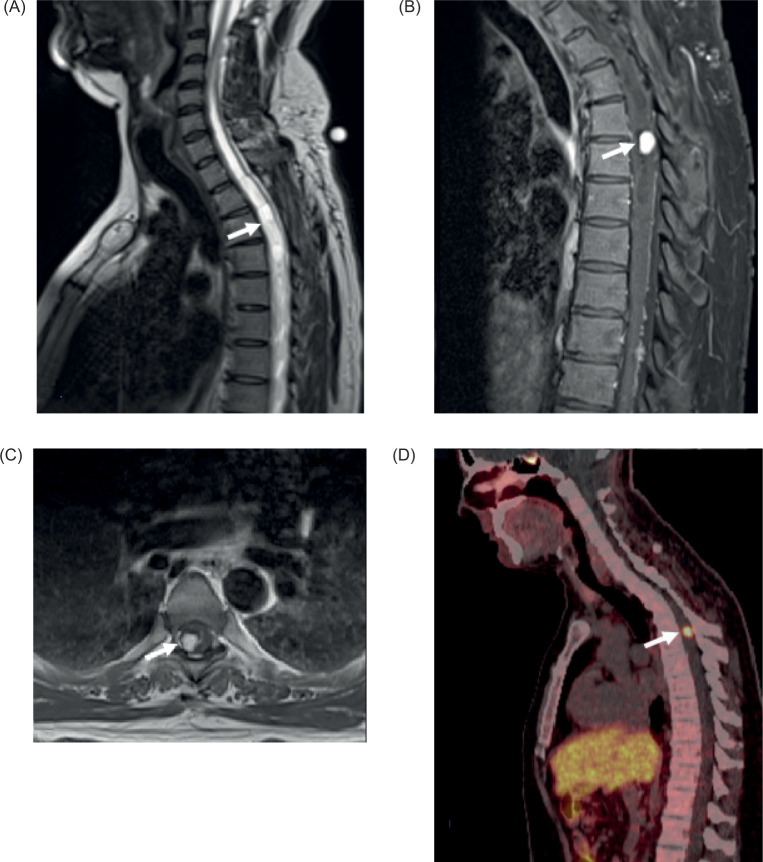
(A) Sagittal T2 image of the thoracic spine shows a long syringohydromyeliain of the cervicothoracic spine (arrow); (B) Sagittal T1 fat sat post contrast and (C) axial T1 post contrast demonstrates an avidly enhancing nodule within the thoracic spine, located within the syringohydromyelia and consistent with a hemangioblastoma in this patient with VHL disease (arrows); (D) Sagittal PET/CT Gallium 68 Dotatatedemonstrate avid uptake of the lesion (arrow).
